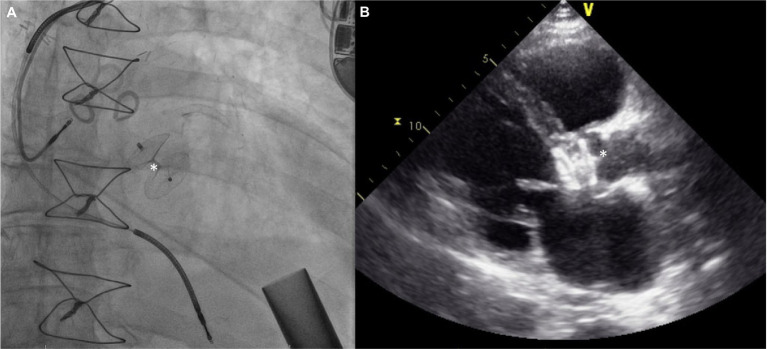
(A) Amplatzer device (^*^) implant for severe AI 3 years after HMII implantation. (B) Echocardiograpic appearance of device.
